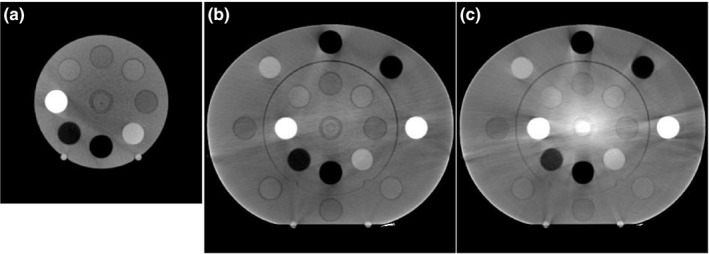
Cone beam computed tomography images acquired using each protocol: (a) S‐scan, (b) M‐scan, and (c) L‐scan
